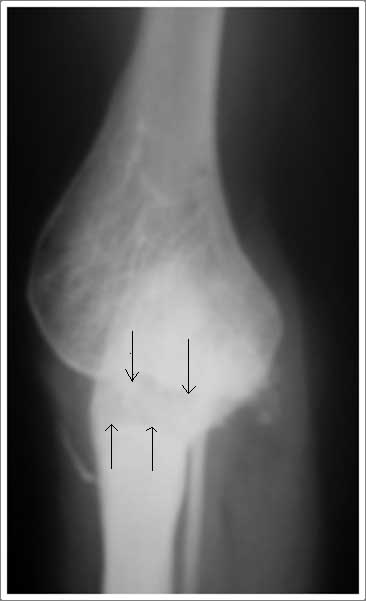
Postoperative antero-posterior radiograph of 28 year female showing the rectangle radiolucent joint line marked by thin black arrows at 80 months follow up.
